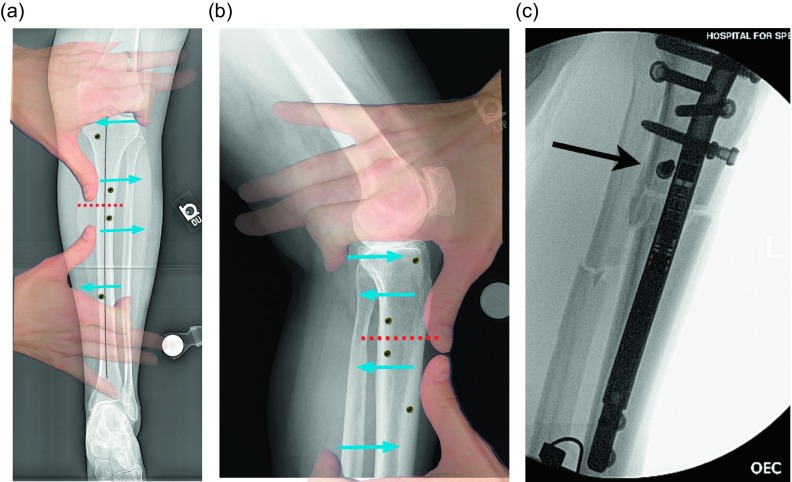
(a) This shows a different patient’s X-rays. The valgus correction is maintained by placing blocking screws in the concavity of the deformity (lateral aspect). Peripheral blocking screws can be used as well. (b) The sagittal plane requires at least one blocking screw in the proximal fragment near the osteotomy (the screw shown at the entry site of the ILN is theoretical and is never used). (c) This lateral film shows a well-placed posterior blocking screw (arrow).Half pins: A half pin is inserted into the proximal tibia using cannulated technique. A K-wire is inserted posterior to the path of the IM nail usually superior and anterior to the fibular head and checked using C-arm. The wire is then over-drilled with a cannulated drill bit through a small incision. The solid half pin (5–6 mm) is then inserted. A second half pin is inserted in the distal tibia, distal to the tip of the ILN. Rotation should be accounted for in this step for later correction (Figure 12).Figure 12. F12:
